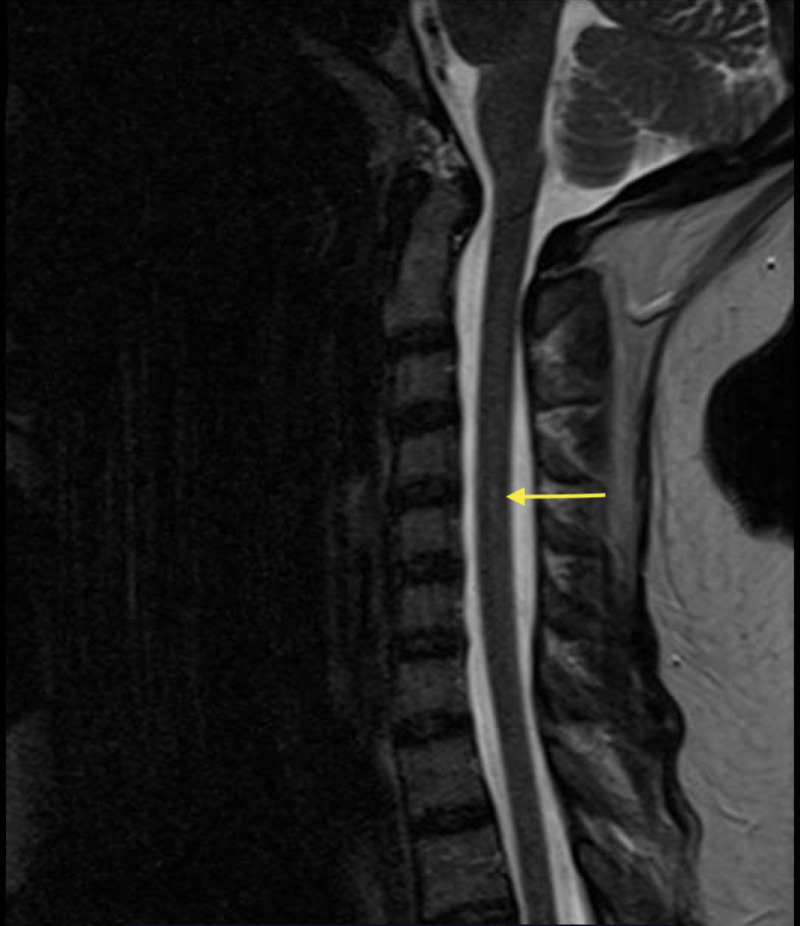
MRI of the cervical spine showing focal central cord T2 signal hyperintensity at C4-C5.
